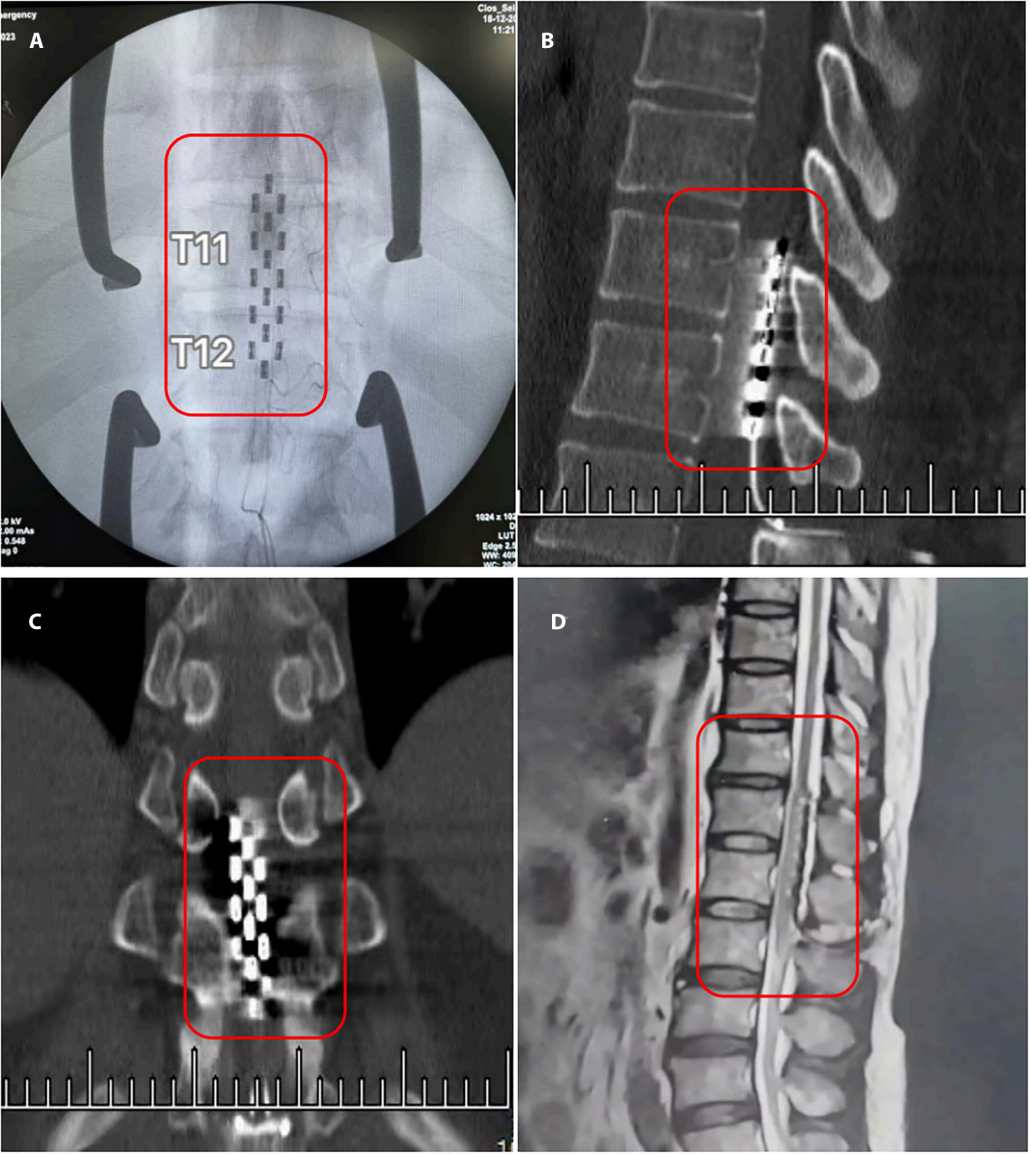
(A) Intraoperative x-ray positioning, taking an example of implantation at T11 to T12. Postoperative computed tomography (CT) in the sagittal (B) and coronal (C) views. (D) Postoperative magnetic resonance imaging (MRI) in the sagittal view.
